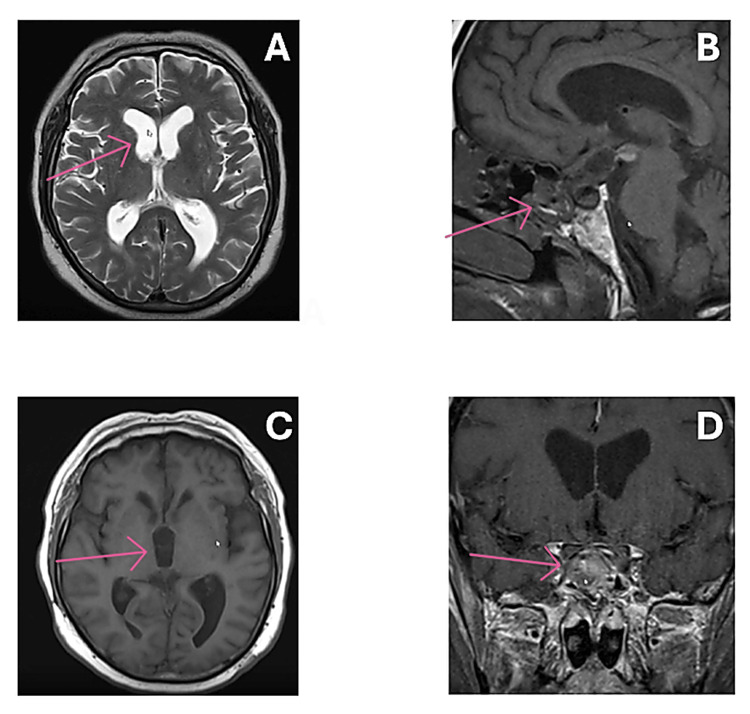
Postoperative MRI scans, approximately 1 month after the second surgical procedure (trans-sphenoidal resection of the craniopharyngioma). Scans show resolution of the cystic component of the craniopharyngioma. Images A and C show the overall resolution of ventricular dilatation, with normalisation of the size of the ventricles, since definitive surgical resection of the tumour. Image B show resolution of the cystic component of the craniopharyngioma in the sagittal plane, in addition to the removal of the suprasellar mass (craniopharyngioma tumour). Image D show the latest MRI scans (coronal plane), following the surgical resection of the suprasellar mass (craniopharyngioma).
